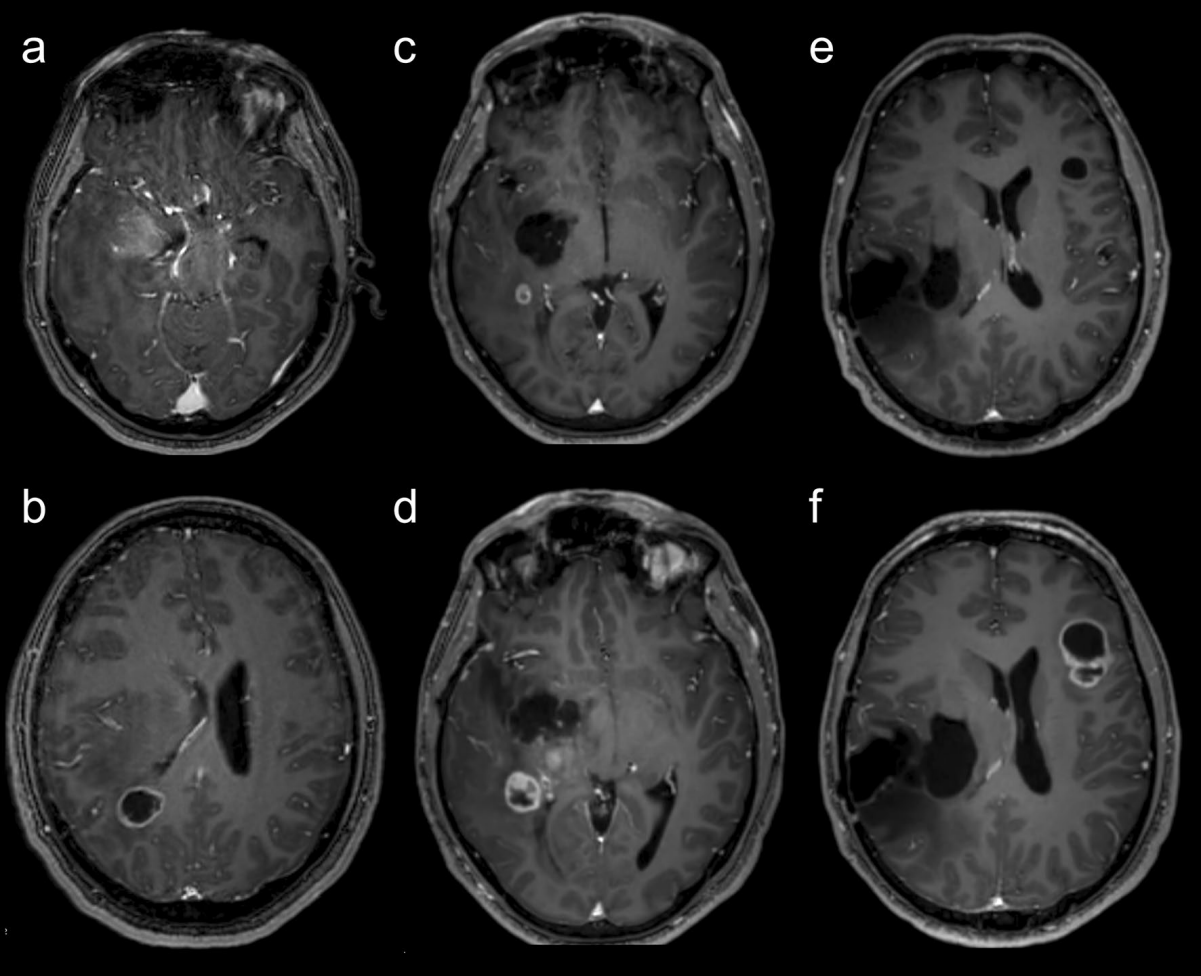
Radiological findings over the course of the patients’ treatment. Axial postcontrast T1 weighted magnetic resonance imaging (MRI) showing a right-sided inhomogeneous contrast enhancing lesion located in the basal ganglia and peritrigonal area (a), as well as a parietal cystoid mass (b). After initial partial resection right peritrigonal tumor progression was seen (c and d). Furthermore, another left frontal rapidly progressive cystic tumor developed (e and f)
